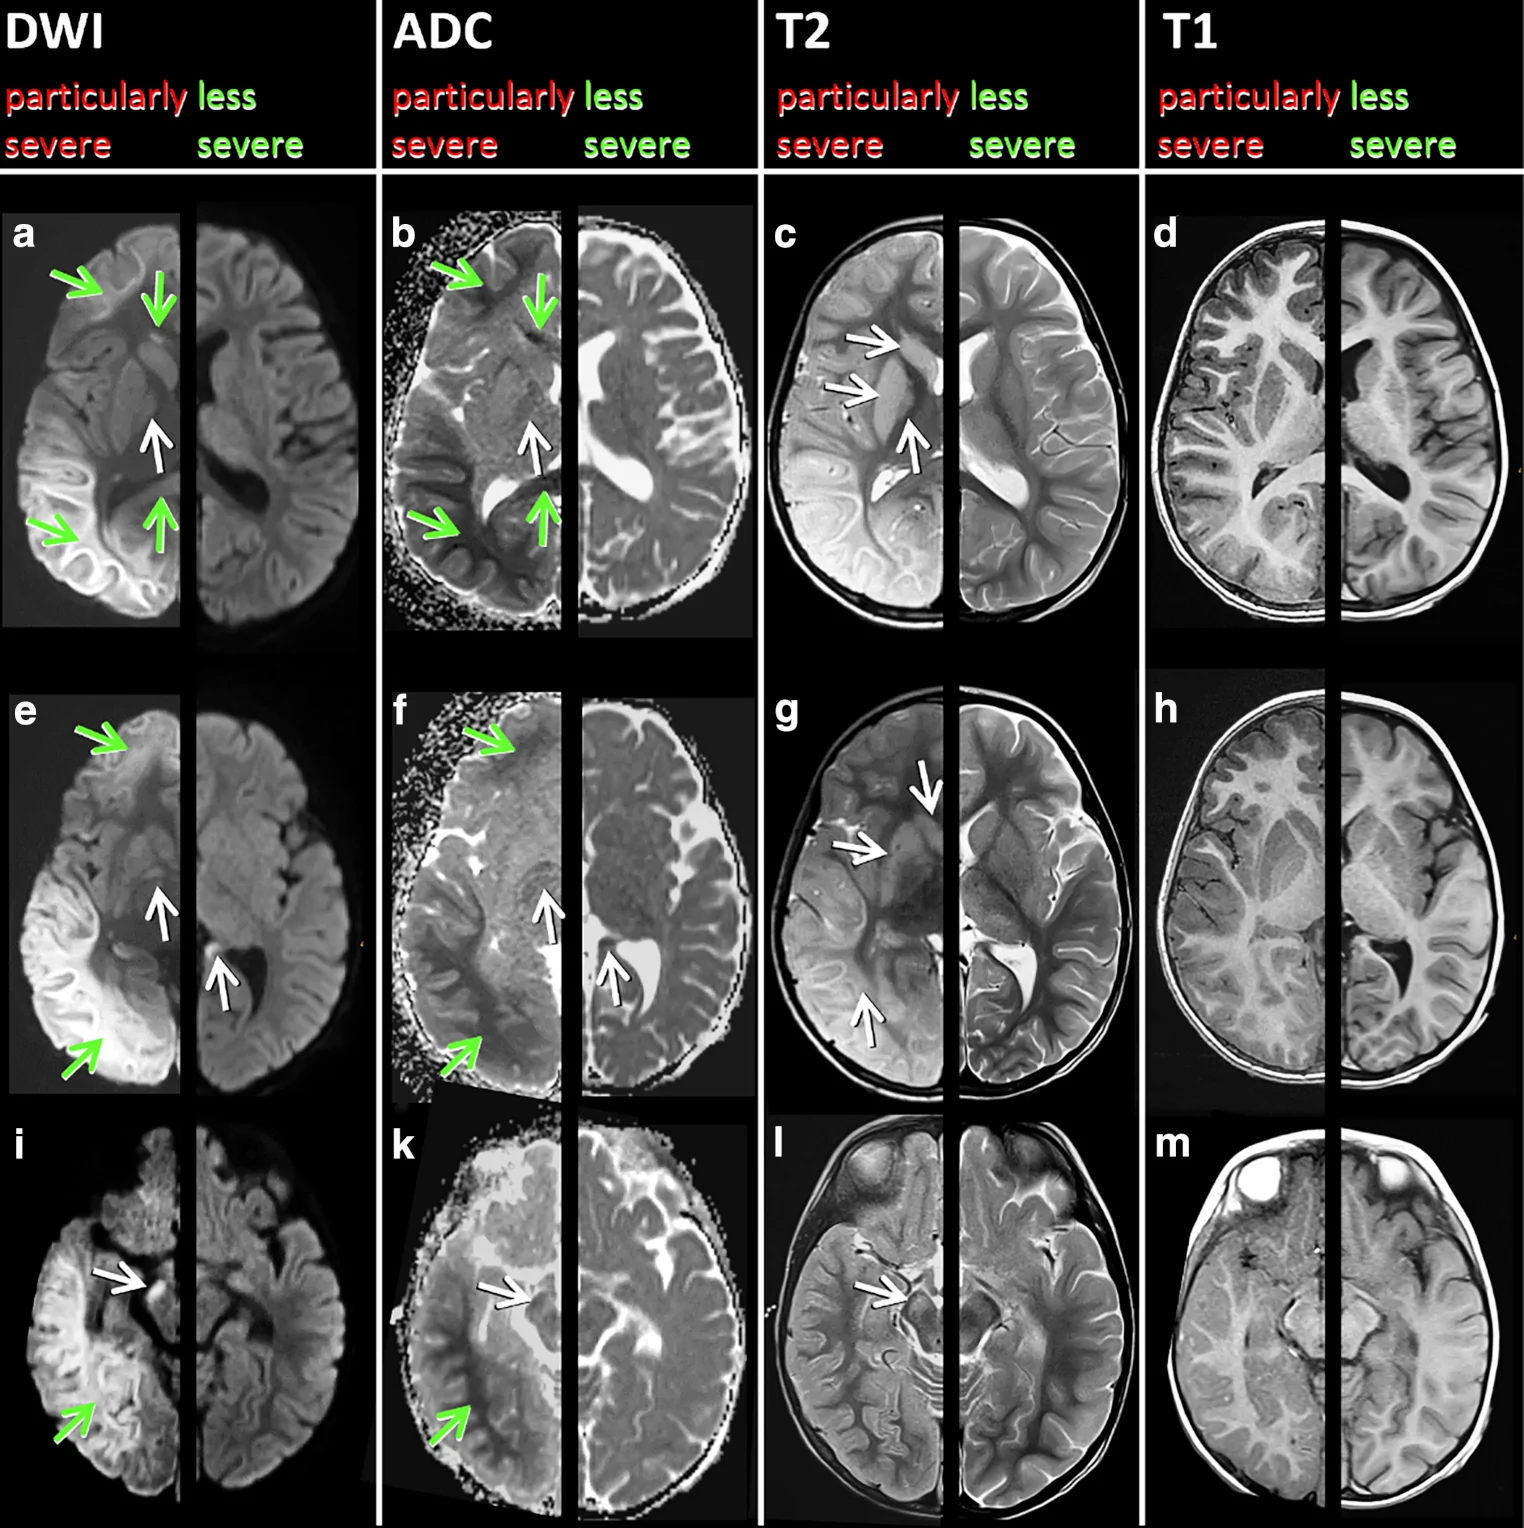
MRI obtained 6–9 days after the anoxic event. Structures with abnormal signal intensities are marked with arrows (green/light gray = absence of these signal alterations predicts less severe outcome). MRI during this time was characterized by a peak in diffusion restrictions in the cerebral white matter (Fig. 4 and 5 bottom). Now, the absence of these diffusion abnormalities in the cerebral white matter predicted less severe outcomes (green/light gray arrows)
